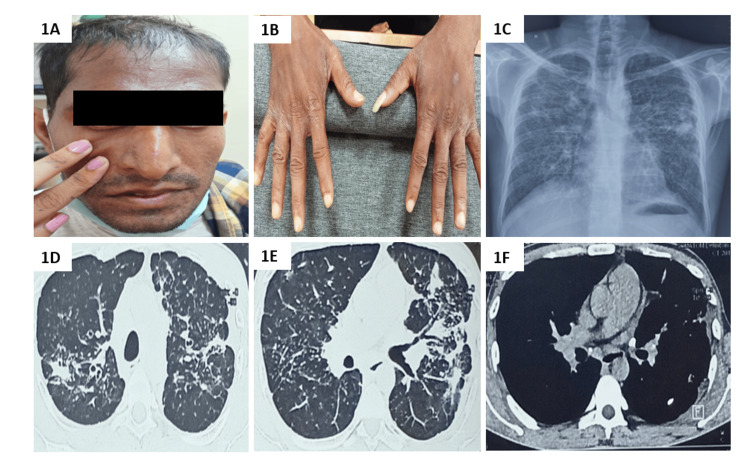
Clinico-radiological findings in Case 1 (A) Positive Ingram’s sign; (B) sclerodactyly of both hands; (C) chest radiograph in posteroanterior projection showing reticulonodular opacities in bilateral upper and mid zones; (D, E) HRCT chest lung window showing septal thickening, fibrotic opacities and conglomerated nodules in bilateral upper lobes; (F) HRCT chest mediastinal window showing calcified mediastinal lymph nodes. HRCT – high-resolution computed tomography
